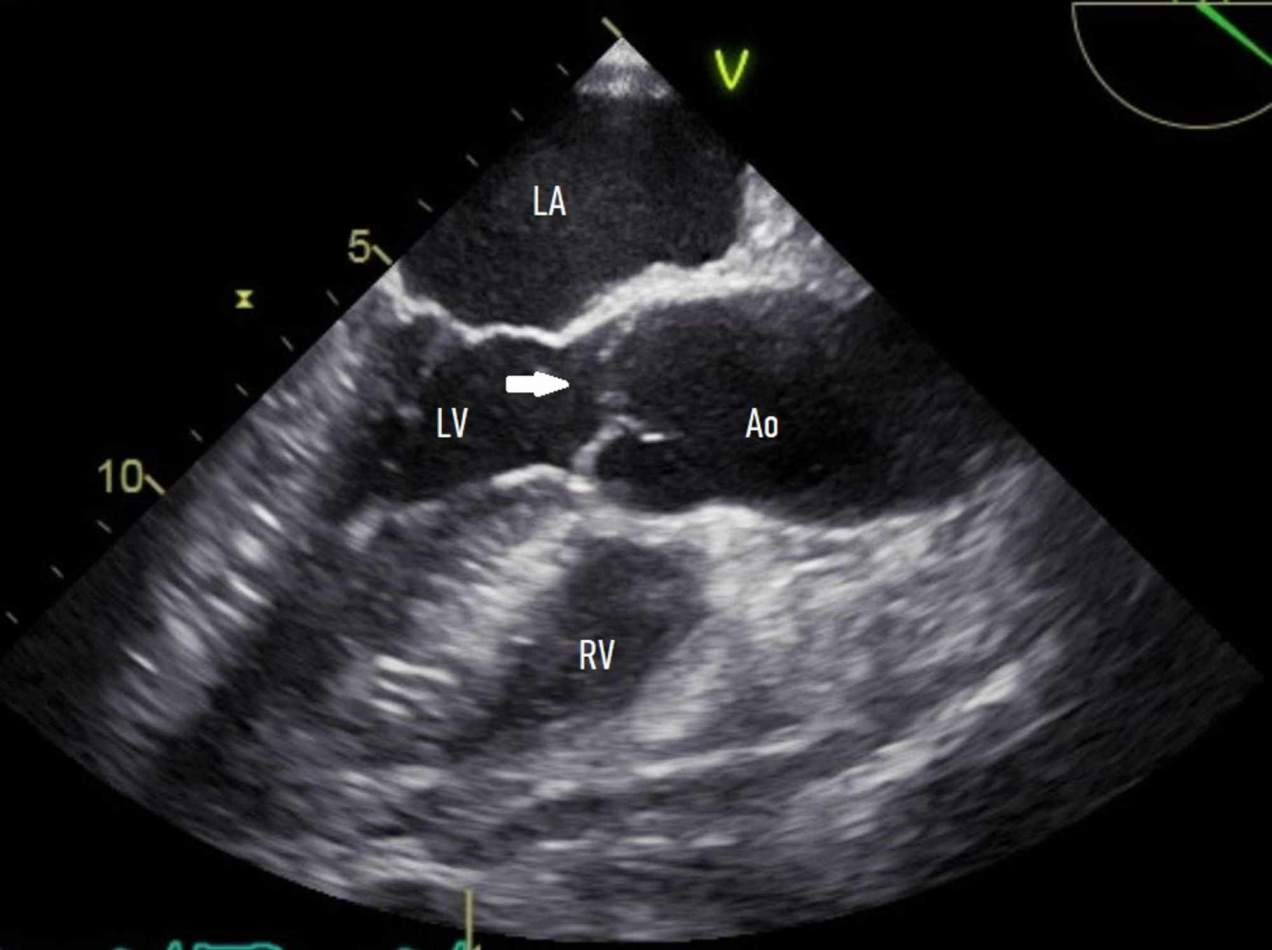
TEE, mid-esophageal long -axis view, showing an approximately 1 -mm echo-density on the ventricular side of the aortic valve (arrow) TEE, transesophageal echocardiogram; LA, left atrium; LV, left ventricle; RV, right ventricle; Ao, aortic root
